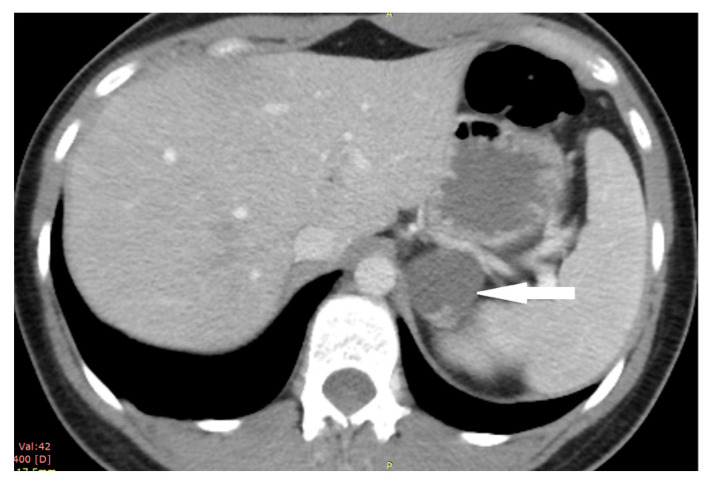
Adrenal lymphangioma. Abdomen CT, transverse cross-section, after eight years.
